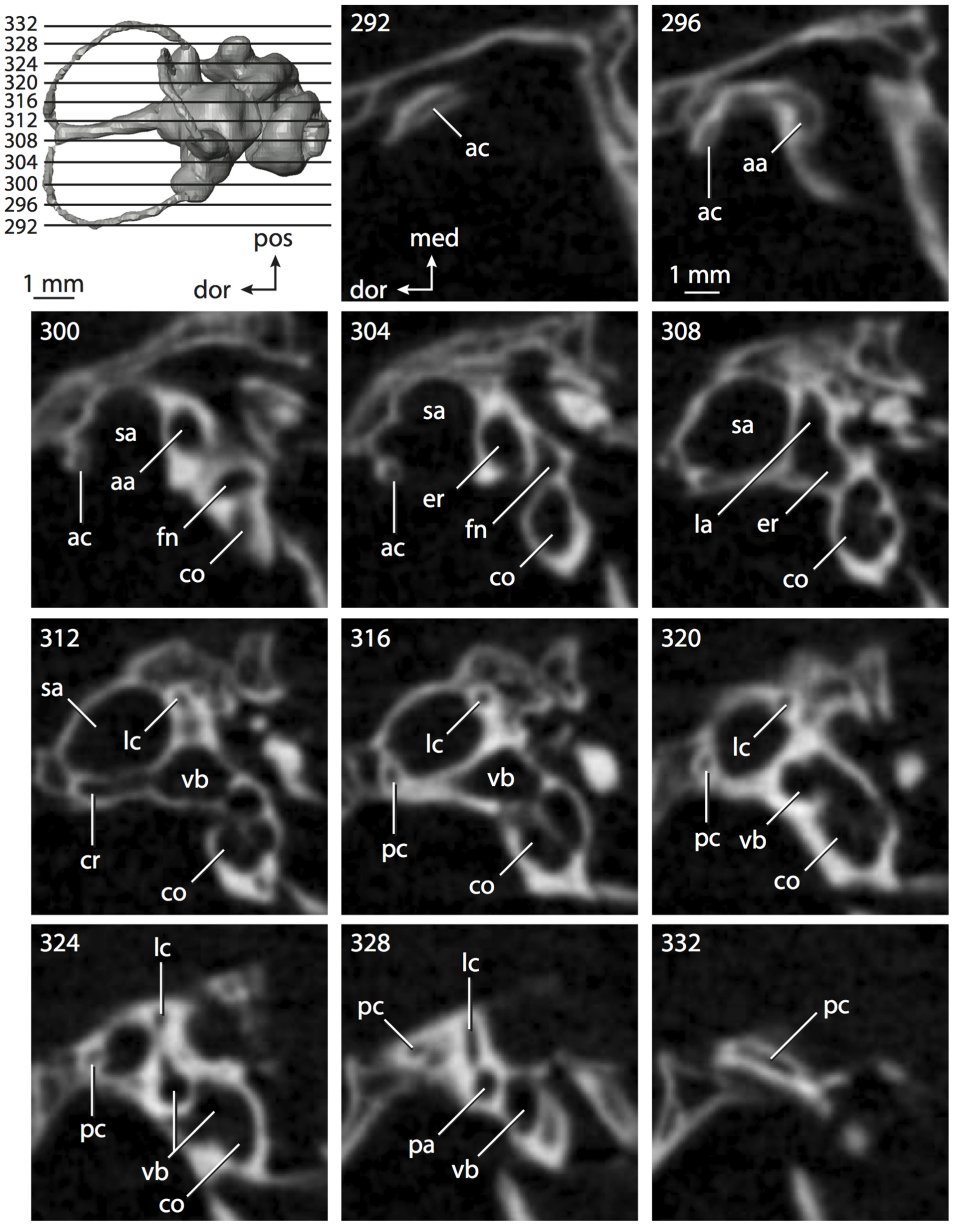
CT slices through ear region of*Hemicentetes semispinosum*. Numbers refer to specific CT slices. Abbreviations listed at the end of the Materials and Methods section.
